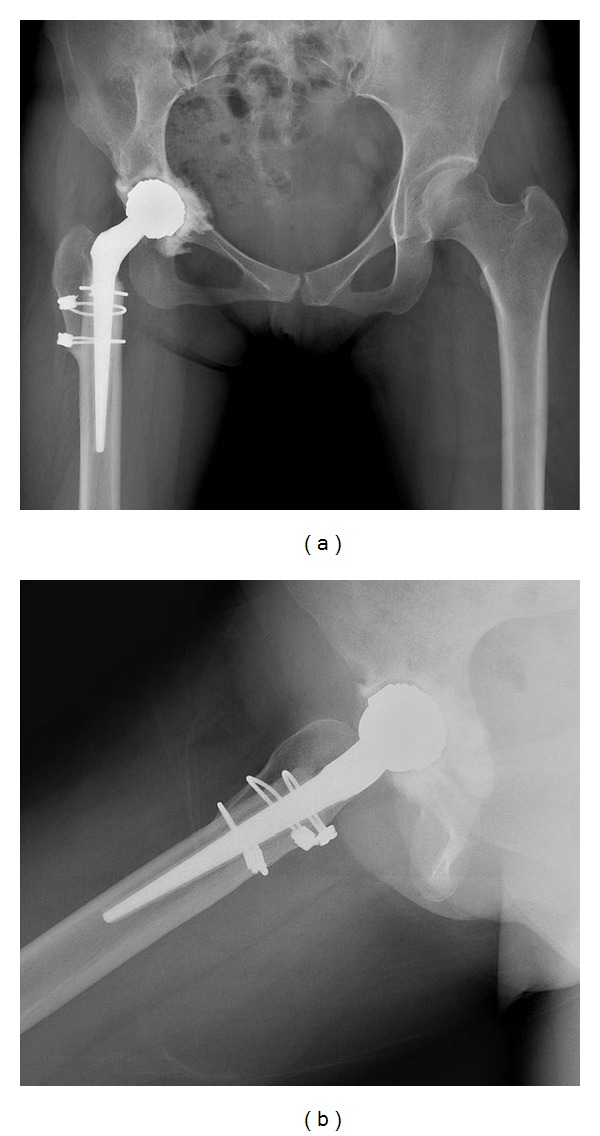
Postoperative radiographs taken 5 years 3 months after THA. (a) Both hip anteroposterior and (b) right hip lateral radiographs show complete bone union at the osteotomy site and no evidence of osteolysis.
